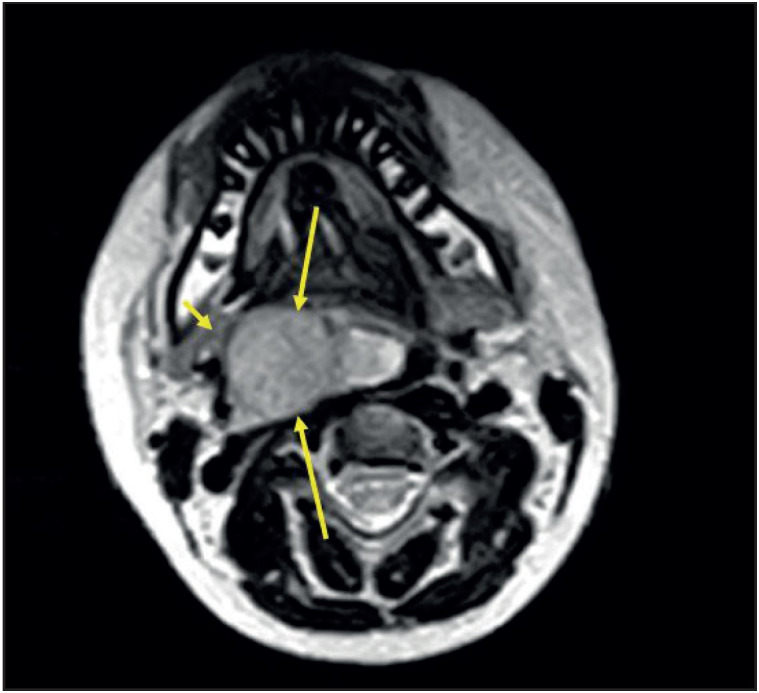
T2-weighted axial MR image shows hyperintense mass lesion within the right parapharyngeal area (long arrows). Maximum diameters in this plane are 24 x 38 mm. The mass is displacing the right submandibular gland (short arrow) but there is no invasion.
